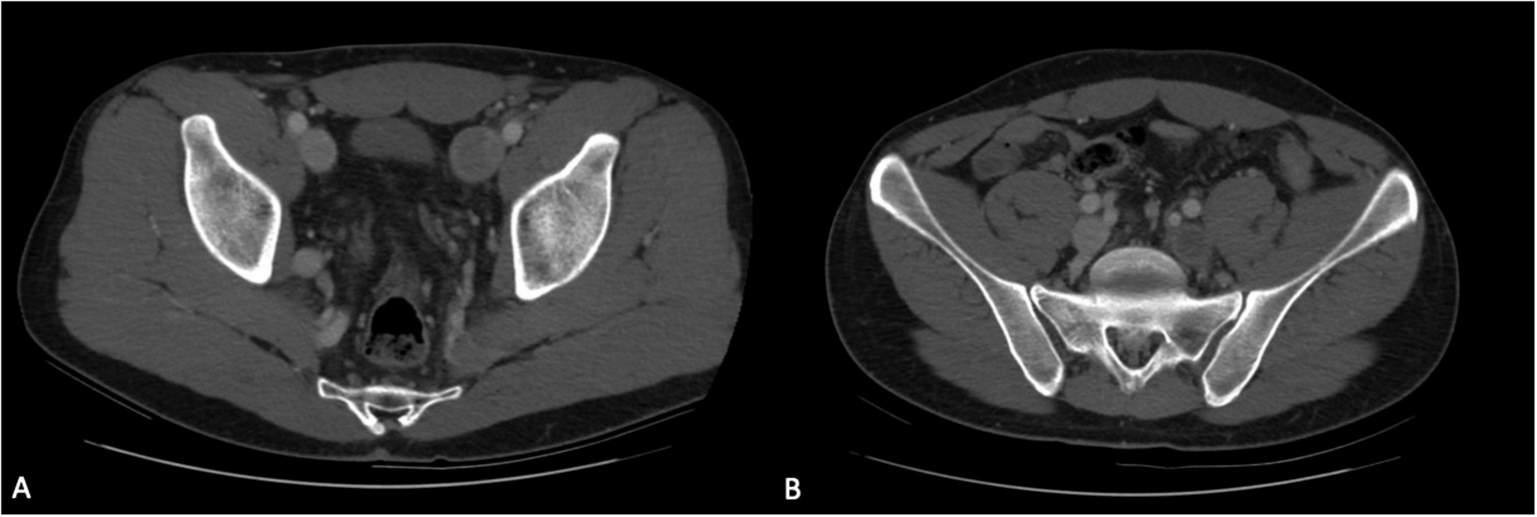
(A,B) Patient's CT-angiography at admission revealing complete thrombosis of left external iliac vein, right internal iliac vein, and partial thrombosis of right external iliac vein.
